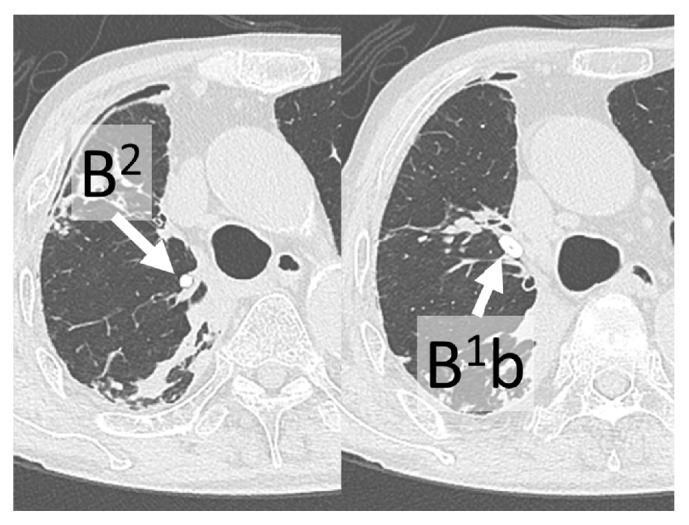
Chest computed tomography shows improvement of pneumothorax and pleural effusion in the right lung. Endobronchial Watanabe spigots were placed in B^2^ and B^1^b of the right lung.
